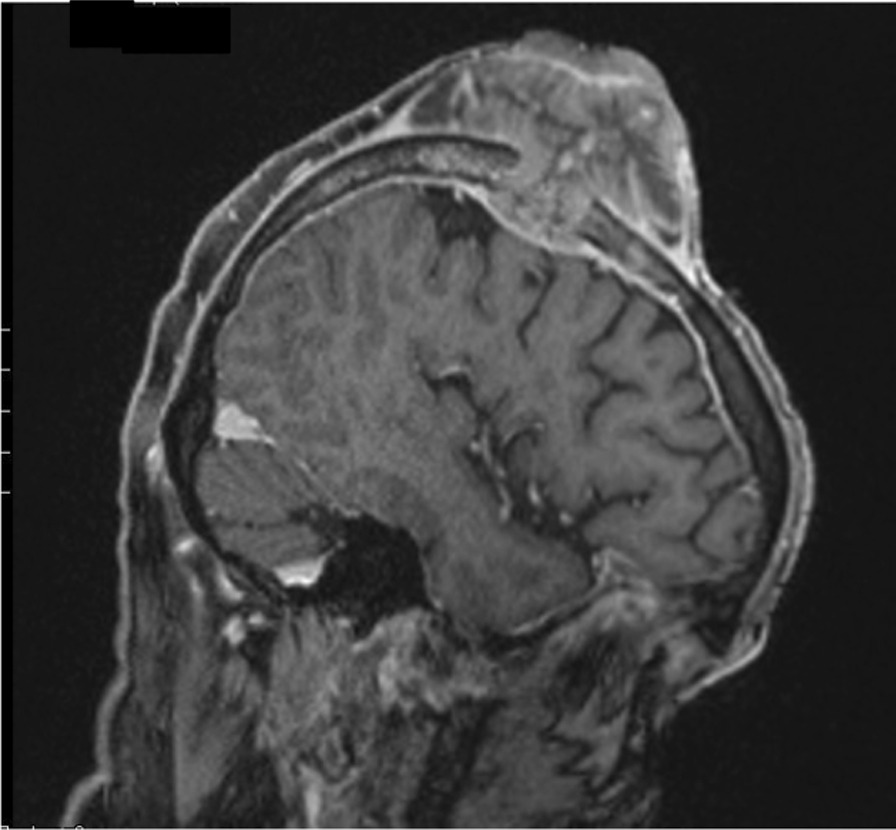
T1 MP-RAGE 3D weighted sagittal contrast-enhanced MRI sequence before definitive radiotherapy. MRI showed a 7.2 × 6.8 × 5.5 cm tumor with destruction of the skull and suspected infiltration of the dura mater and superior sagittal sinus. Biopsies showed cutaneous squamous cell carcinoma. The TNM stage was cT4a cN0 cM0, stage IVA using the Union for International Cancer Control (UICC) staging system (8th edition).
